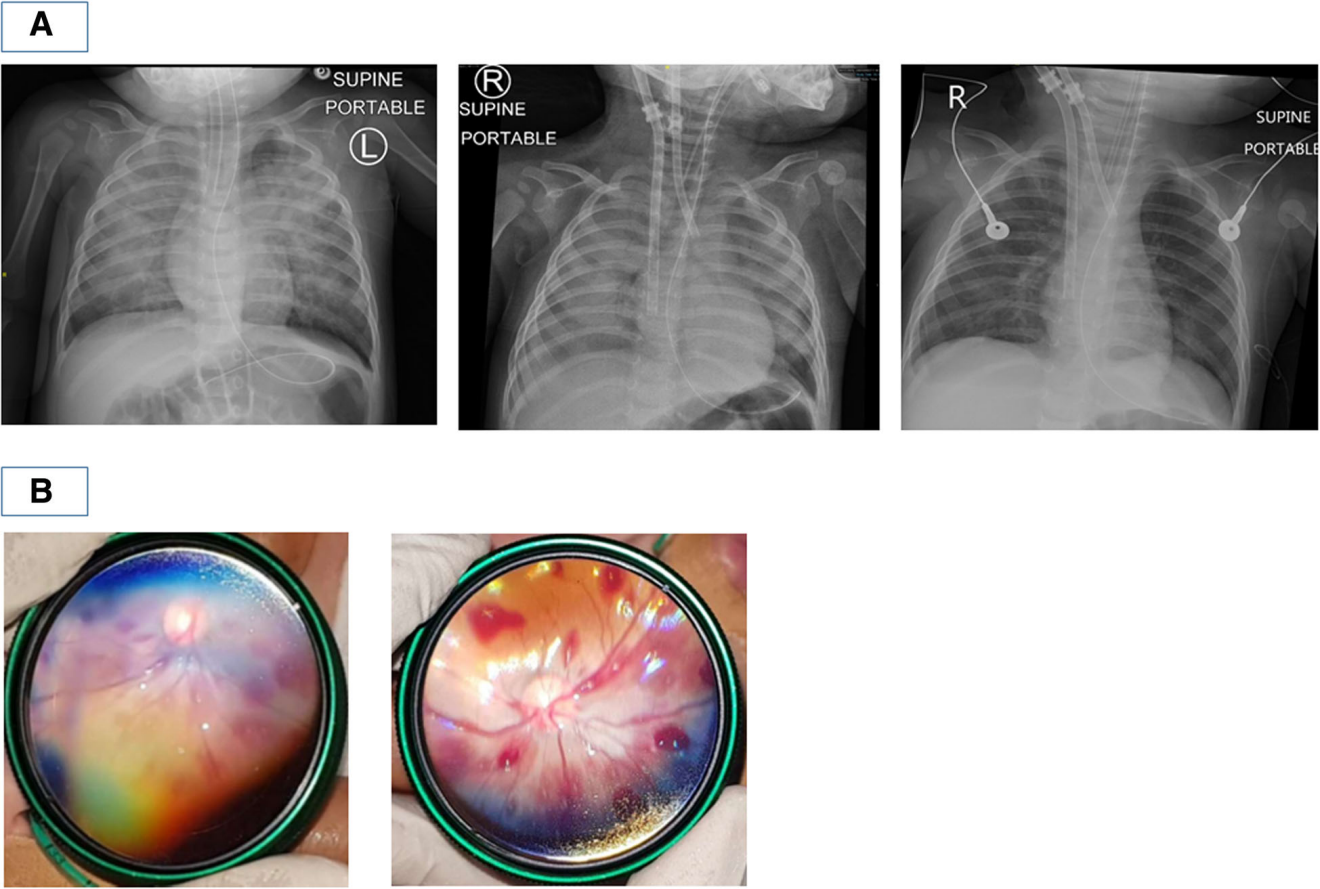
a Serial Chest X-rays done showing rapid reversal of pulmonary oedema: leftmost image: done in Children’s Emergency after change of endotracheal tube showing radiographic findings of pulmonary oedema; middle image: done immediately after ECMO cannulation; rightmost image: done the following day, less than 24 h after initiating ECMO. b Slit-lamp examination showing extensive acute bilateral intra-retinal haemorrhages; left image: left retina; right image: right retina
